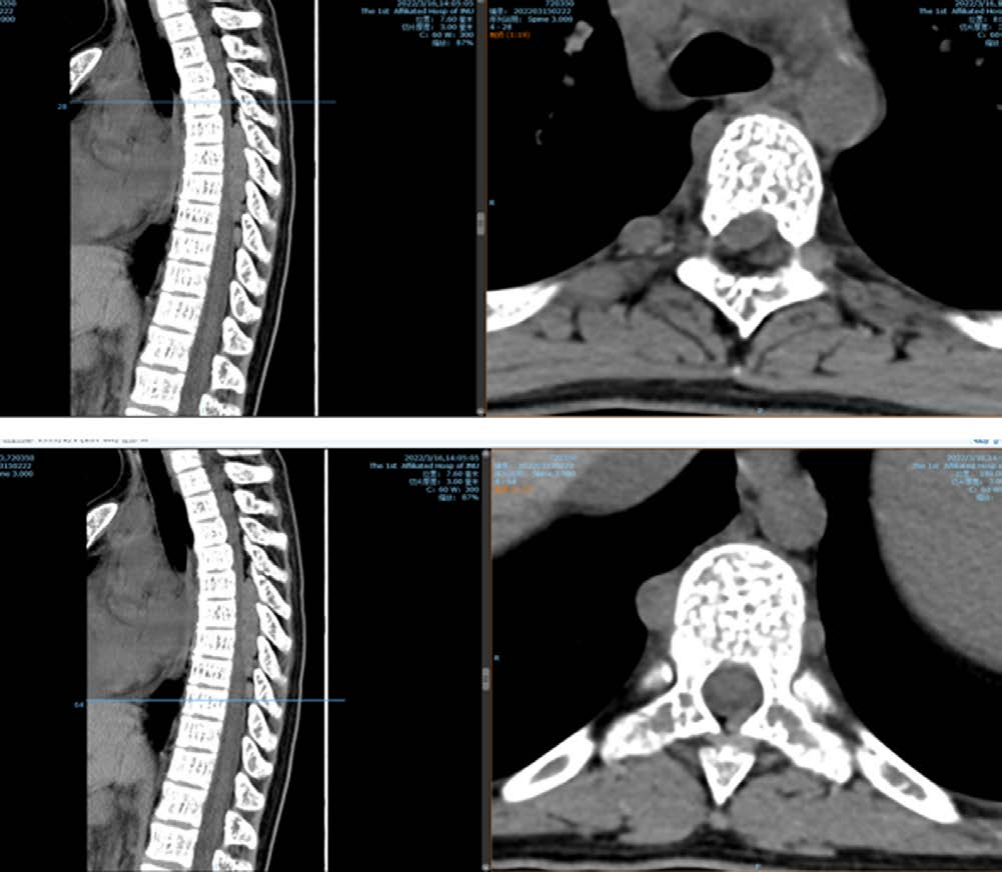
Case 2: preoperative thoracic spine X-ray.
